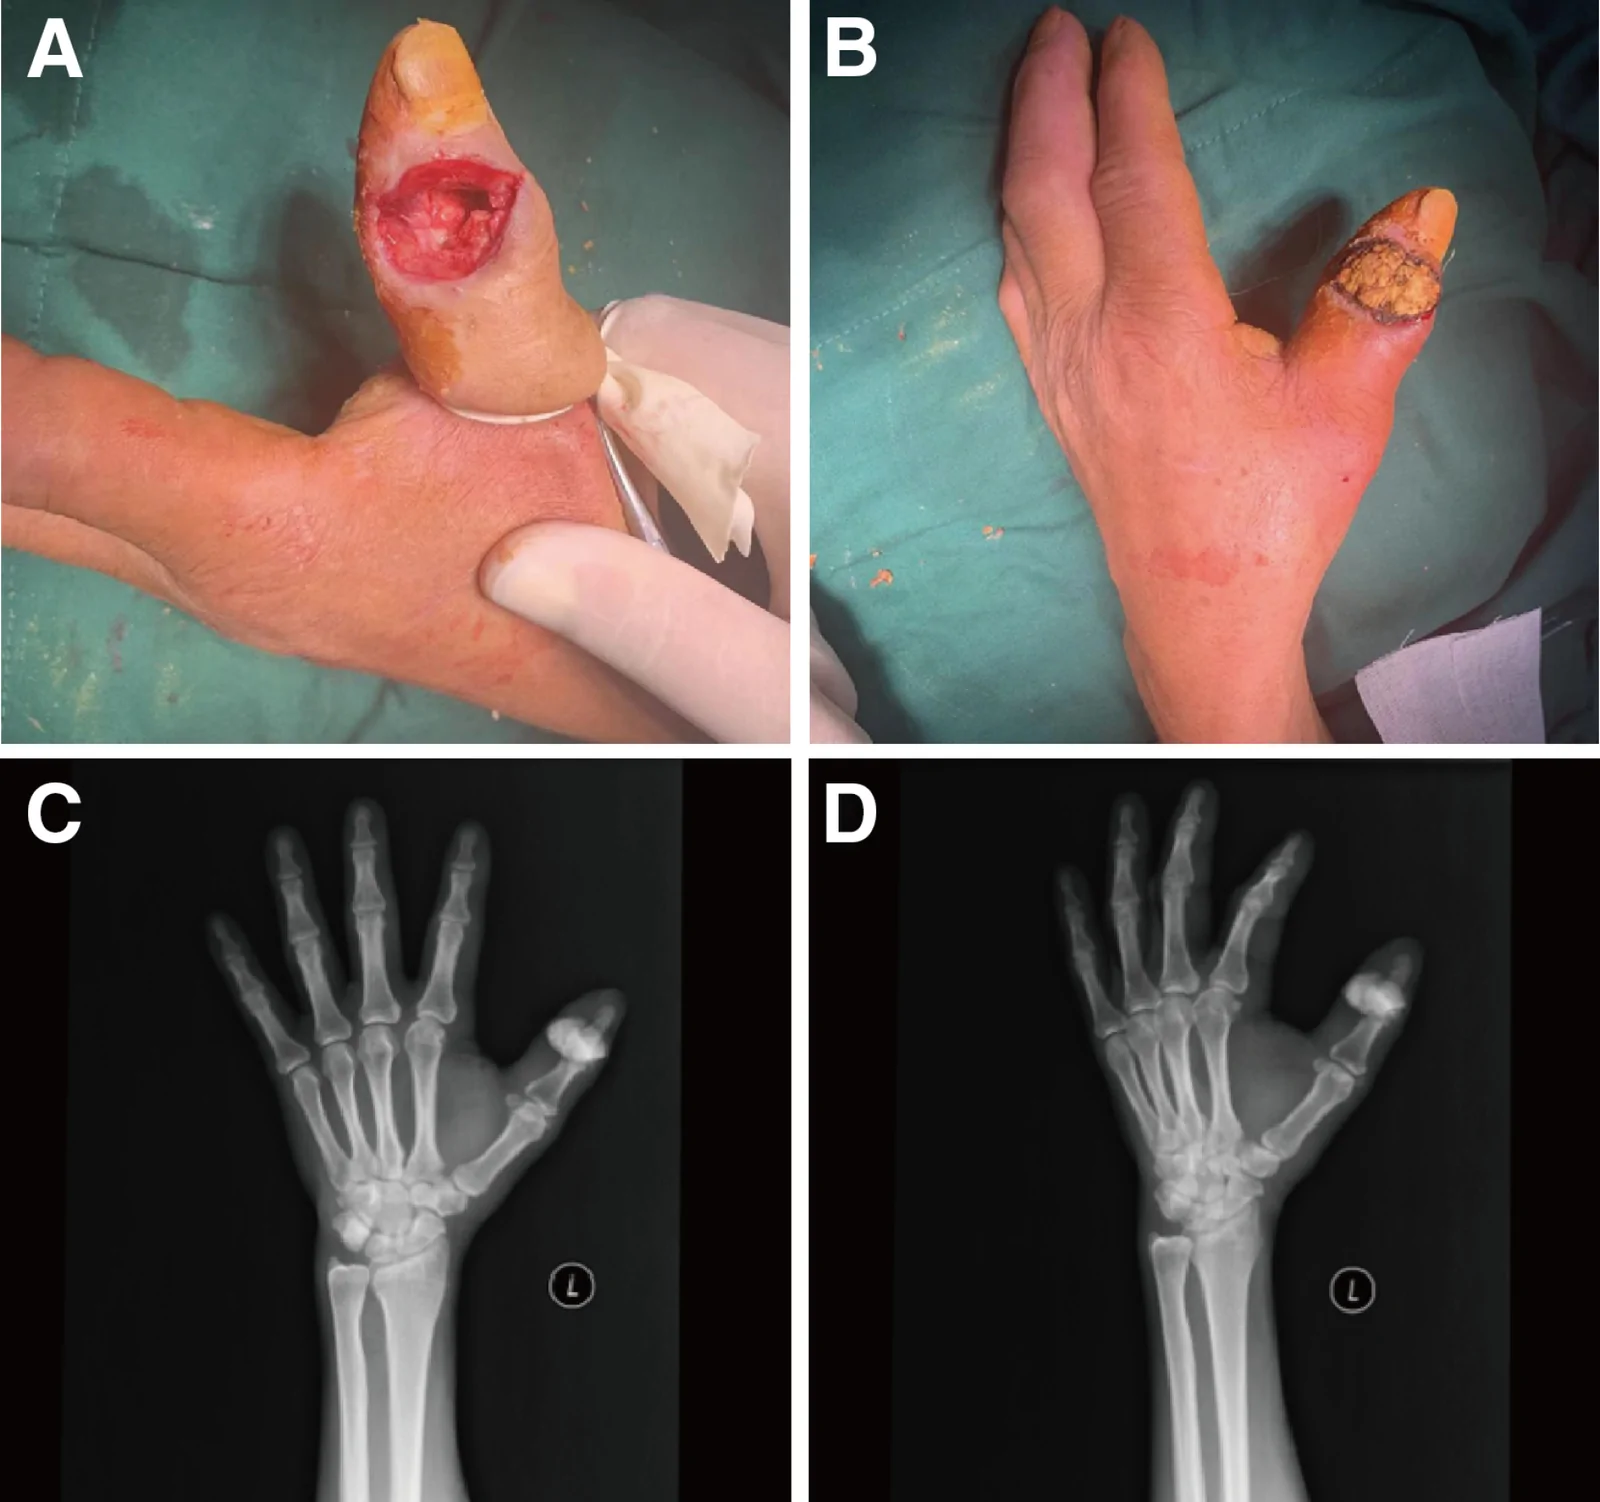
Preoperative (A) and postoperative (B) photos of debridement and bone cement filling treatment for necrotic tissue on the left thumb. Postoperative anteroposterior (C) and lateral (D) X-rays of the left hand.
